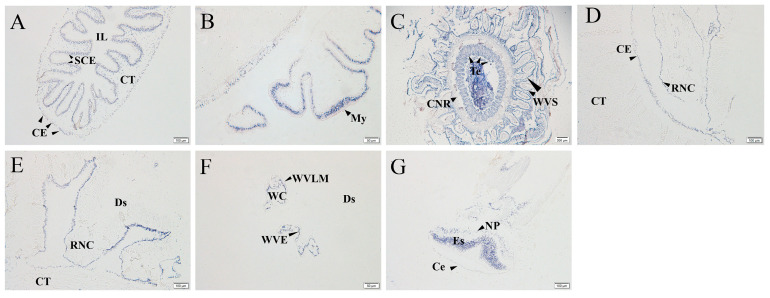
Localization of holotocin precursor in *A. japonicus* using mRNA in situ hybridization. (A) Intestine. (B) Mesentery. (C) Oral position: water vascular system, tentacle and circumoral nerve ring. (D) Longitudinal muscle. (E) Body wall. (F) Water canal. (G) Tube feet. Ce, cuticle; CE, coelomic epithelium; CNR, circumoral nerve ring; CT, connective tissue; Ds, dermis; Es, epidermis; IL, intestine lumen; My, mesentery; NP, nerve plexus; RNC, radial nerve cord; SCE, simple columnar epithelium; Te, tentacle; WC, water canal; WVS, water vascular system; WVE, water vascular epidermis; and WVLM, water vascular longitudinal muscle. Holotocin precursor signals in different tissues were shown by arrows. Scale bar: 200 µm in (C); 100 µm in (A,D,E,G); 50 µm in (B,F).
